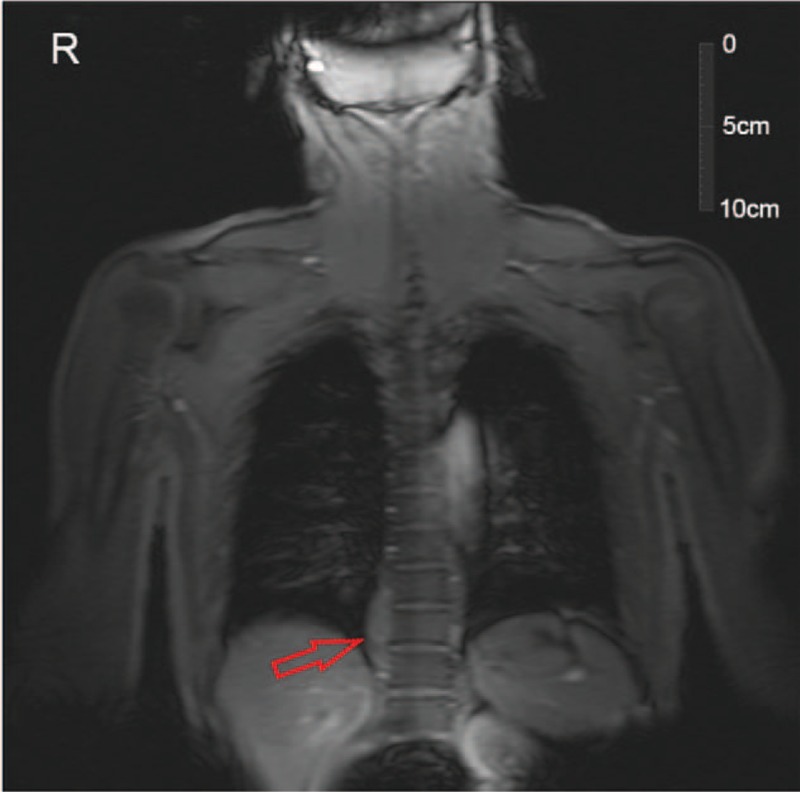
Magnetic resonance imaging (coronal) showed lesions near centrums 9–11 of the thoracic vertebrae (red arrow).
